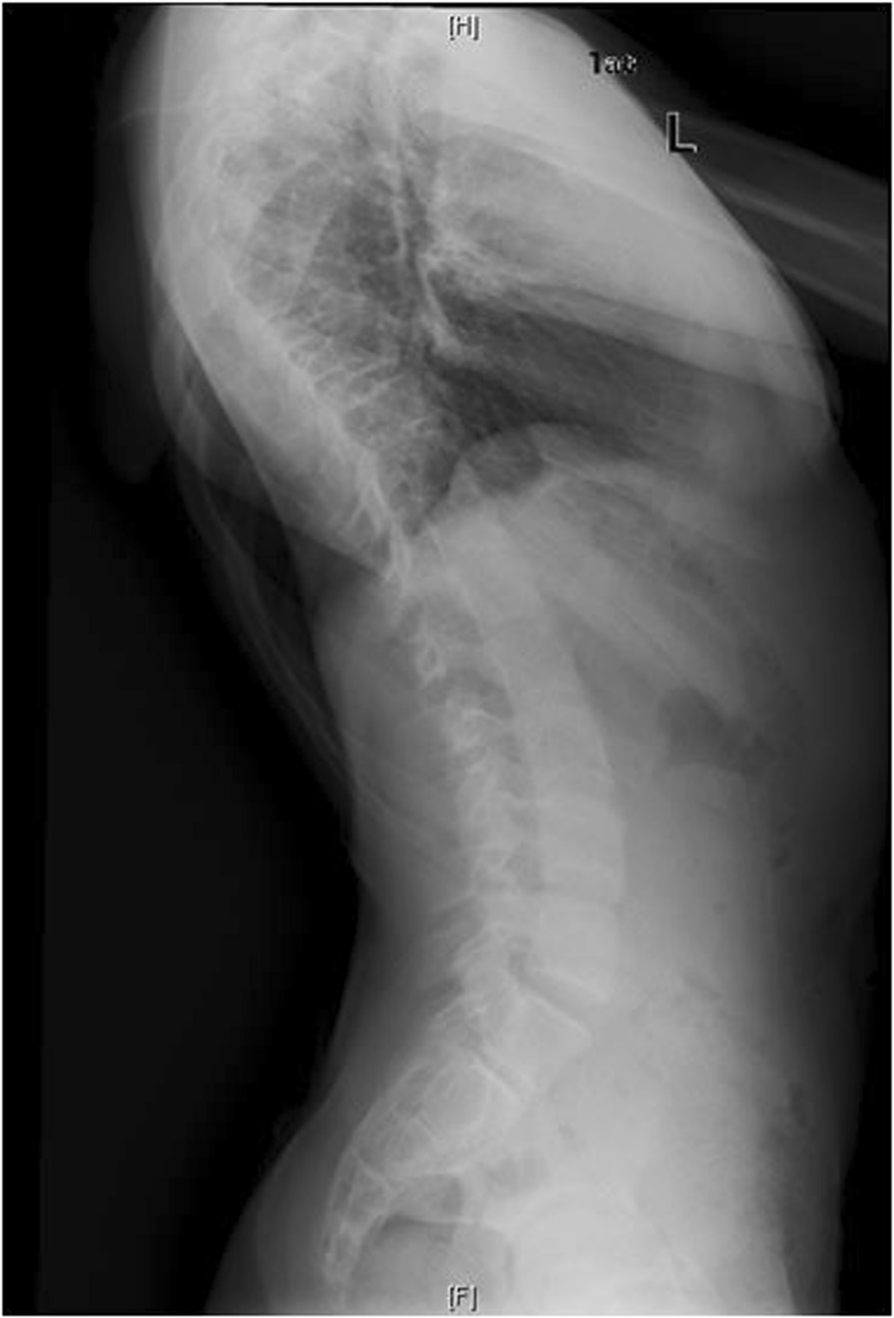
Preoperative radiograph of 12 year-old female with NF.
